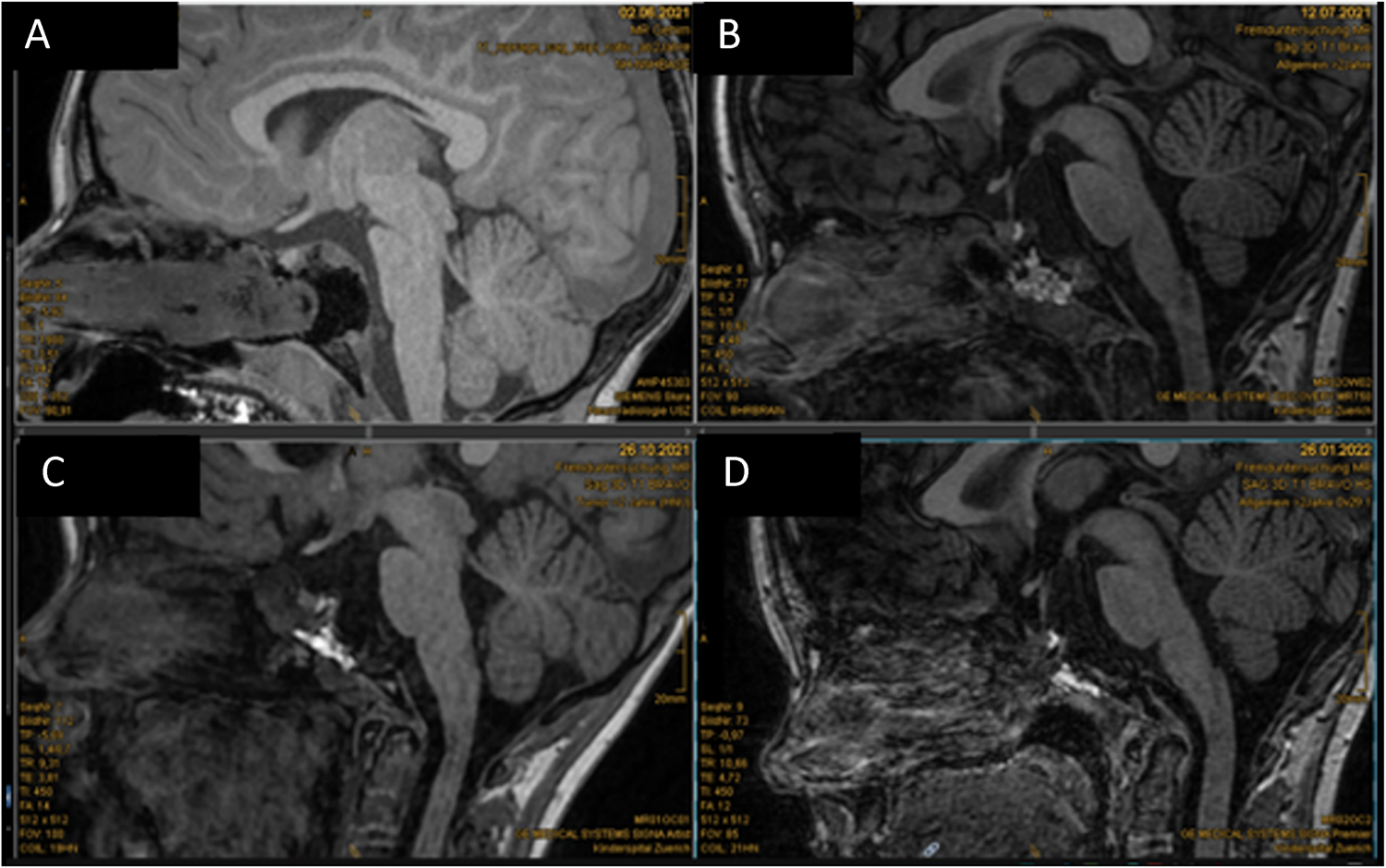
MRI results in patient 3 A intraoperatively, B 1 month postoperatively. C 5 months postoperatively, and D 8 months postoperatively, showing no swelling of the patch or signs of infection. Slow resolving of fat patch can be observed
